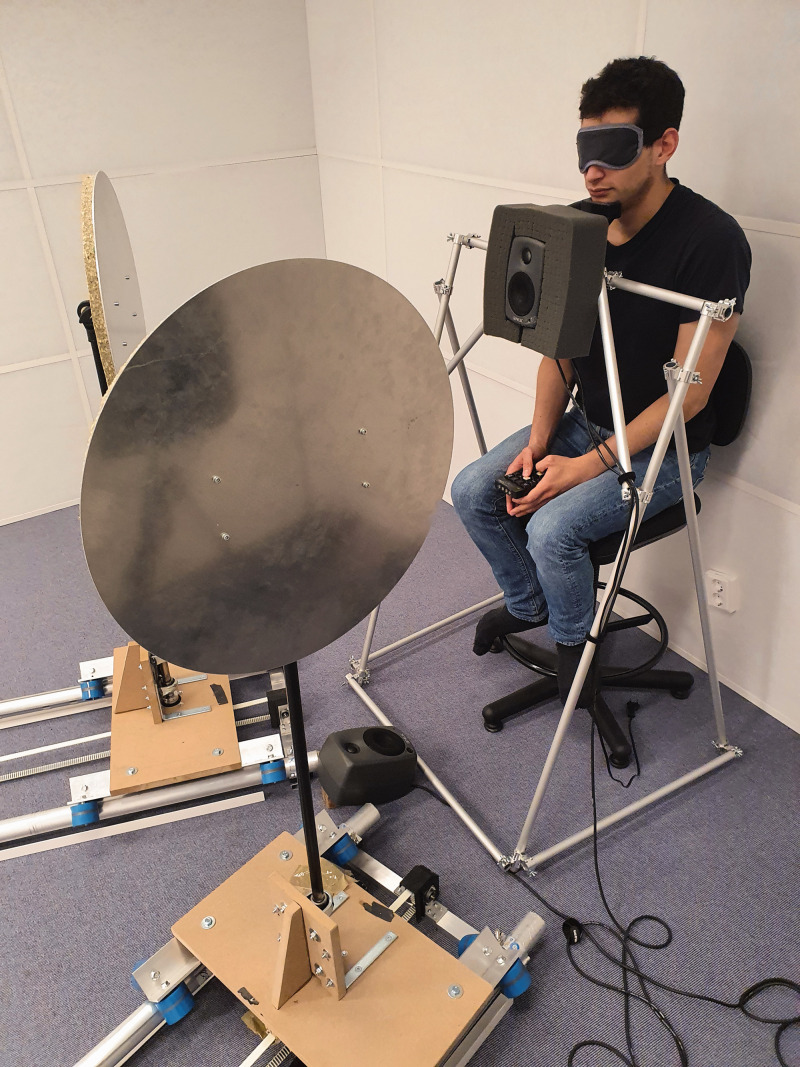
The Echobot. Blindfolded participant (first author, CT in the picture) sitting with his chin fixed behind the loudspeaker and in front of the Echobot. The loudspeaker was located 1 m away from the Echobot at a height of 1.20 m. The masking noise was played from the loudspeaker on the floor in front of the participant. The participant gave his or her responses using a small wireless numerical keyboard. Notice that the distance between the listener and reflective disks varied throughout the experiment; the distance to the disk shown in the figure is only 1 of 10 distances included in the experiment.
